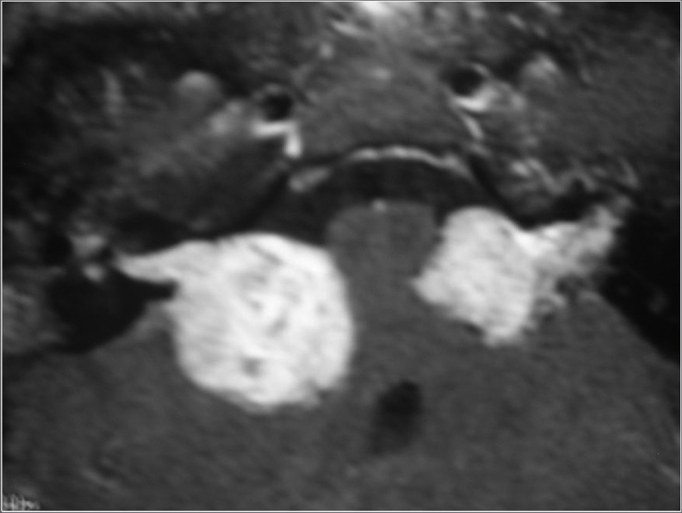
MRI (T1 contrast-enhanced) case 1 preoperative axial view.
